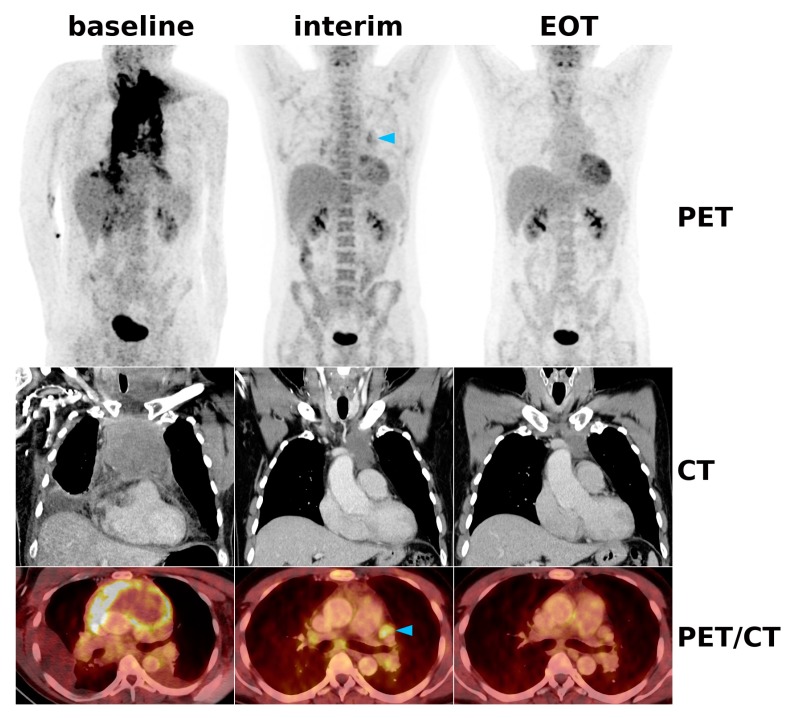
A 44-year-old patient with DLBCL involving the mediastinal, hilar, and lower neck lymph nodes. At interim restaging after three cycles of R-CHOP, the initially strong [18F]FDG uptake had dramatically decreased in all nodal regions, with only some hilar and mediastinal lymph nodes (blue arrowhead) still showing slightly higher uptake than the liver (i.e., Deauville 4 with reduced uptake), which fulfills the Lugano criterion for PR. Since the size of the lymph nodes, including the dominant bulk in the mediastinum, had clearly decreased by >30%, this also represented PR according to RECIL. At EOT, the [18F]FDG uptake was now lower than that of the liver and mediastinal blood pool (i.e., Deauville 2), fulfilling the CR criteria of both Lugano and RECIL.
